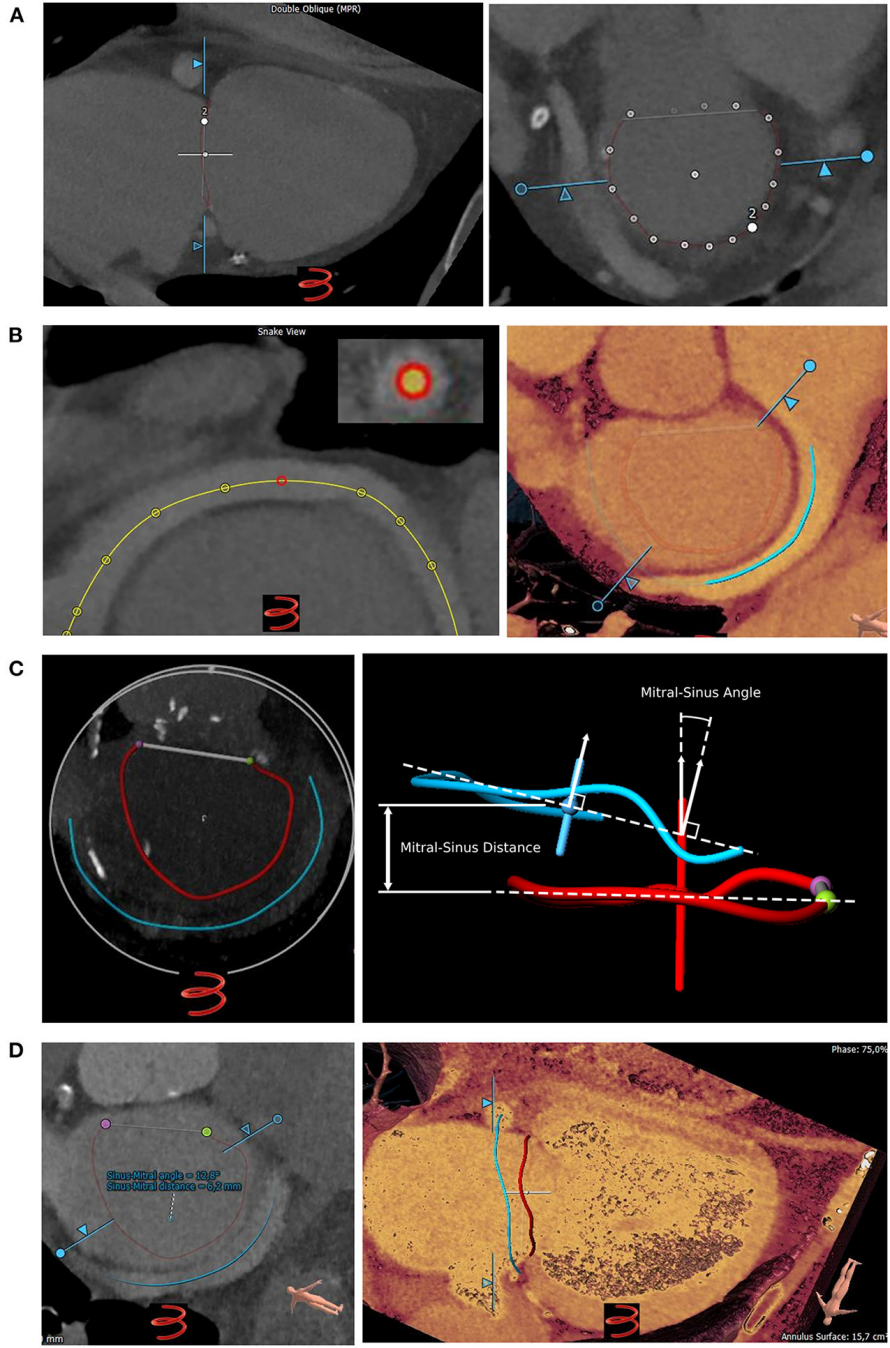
Assessment of coronary sinus to mitral valve annulus topography using a Multislice CT (MSCT) analysis prototype software. (A) Left: Semiautomatic tracking of the mitral valve annulus (MVA). Right: White dots indicate the tracked MVA. (B) Left: Tracking the coronary sinus (CS; yellow line). Right: MSCT showing the CS (blue line) entering the right atrium. Red line: MVA. (C) Left: 3D-model of MVA (red line, D-shape) and CS (blue line) showing their anatomical relation. Right: Centroid (red dot for MVA and blue dot for CS) and axis (red line for MVA and blue line for CS) of the MVA and CS. The normal vector is visualized by the horizontal dotted line. The sinus-mitral angle is located between the axes/normal vectors of the MVA and the CS. (D) Left: After MVA and CS reconstruction the prototype software calculates both distance and angulation of CS to MVA planes automatically. Right: Three-dimensional visualization allows evaluation of CS to MVA topography.
